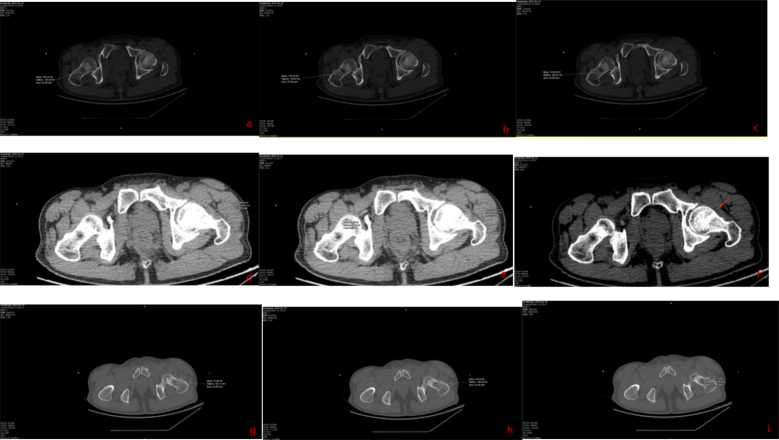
Retrospective CT attenuation measurements were performed around the hip joint.
